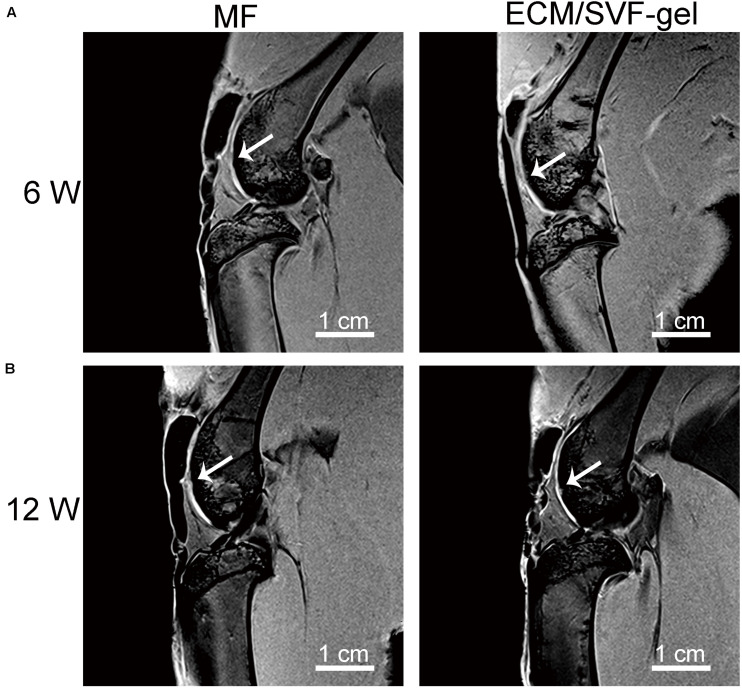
High-resolution MRI of repaired knees. Representative MRI images at 6 weeks (A) and 12 weeks (B) post-operation. White arrow, repaired sites of articular cartilage; scale bars = 1 cm.
